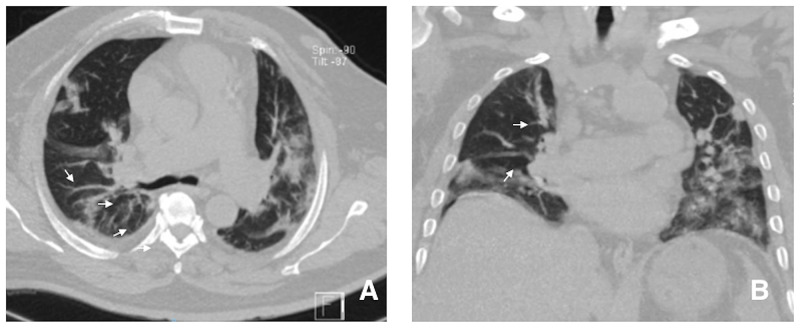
CT images of the chest compatible with the Macklin effect. Ground-glass images and linear air collections indicative of air leak (arrows).
